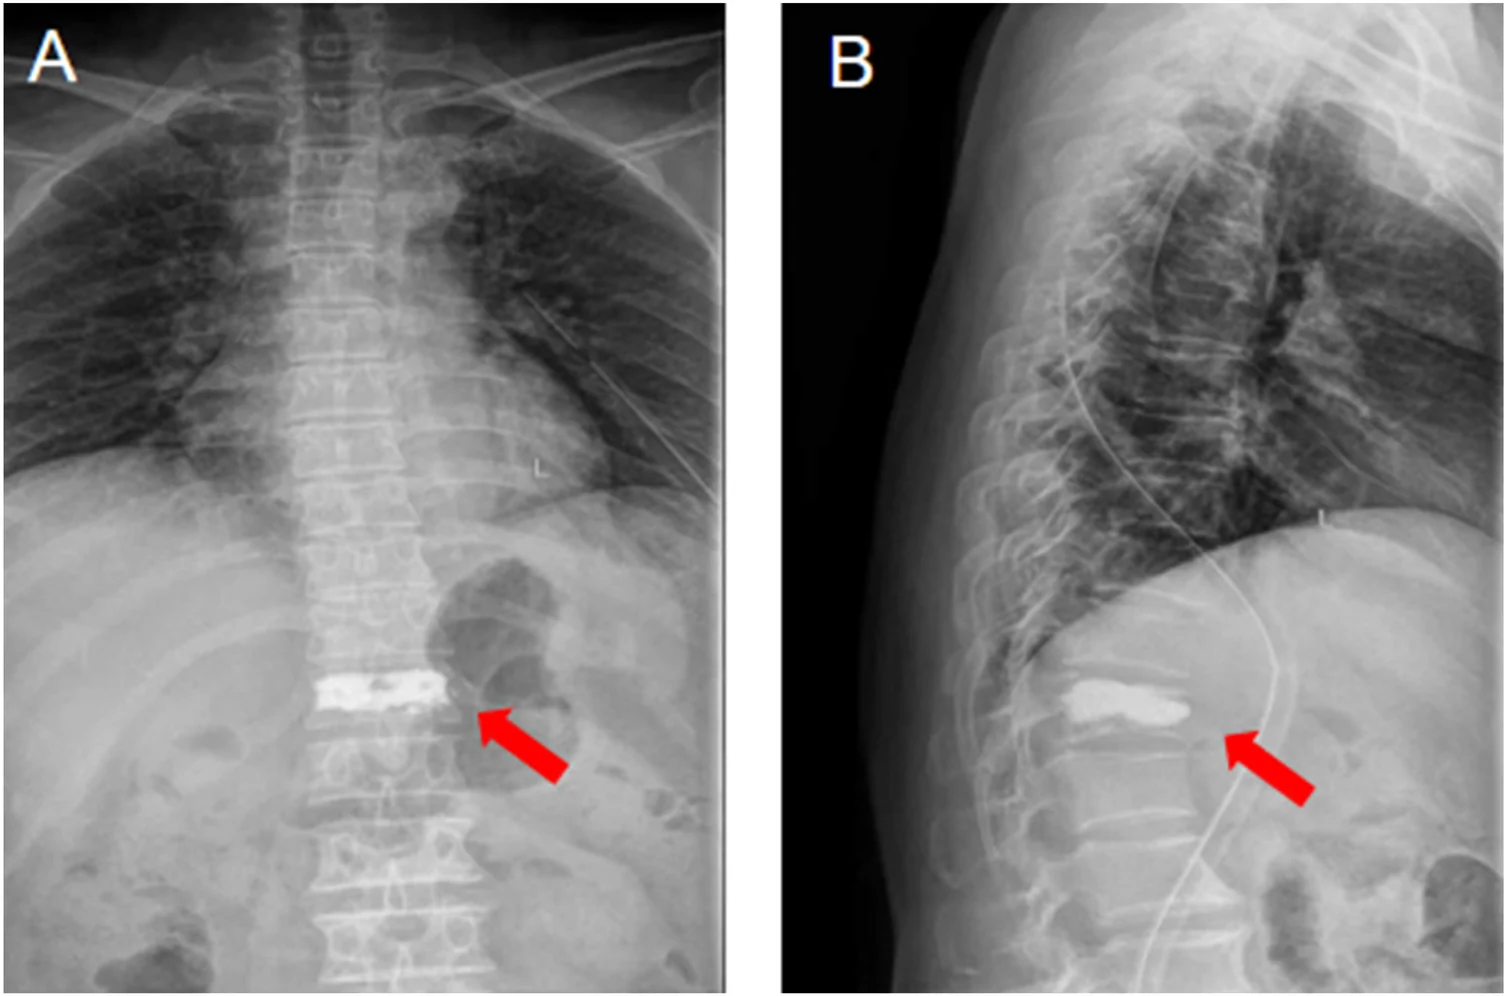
Postoperative anteroposterior (A) and lateral (B) thoracolumbar plain radiographs after PKP, showing satisfactory cement distribution and restored vertebral height.
